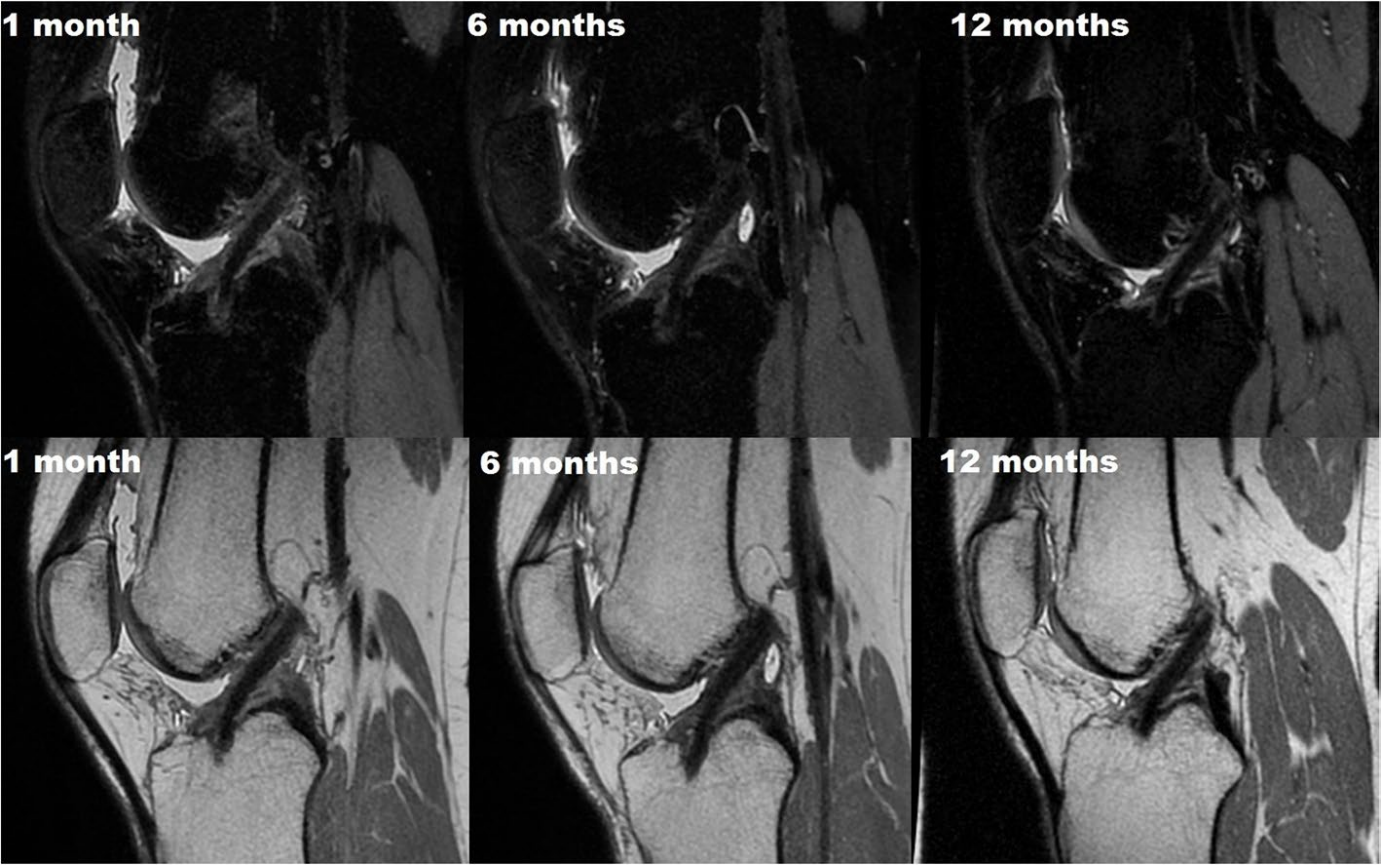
MRI of hamstring ACL graft that was harvested preserving the distal portion 1, 6, and 12 months after surgery
